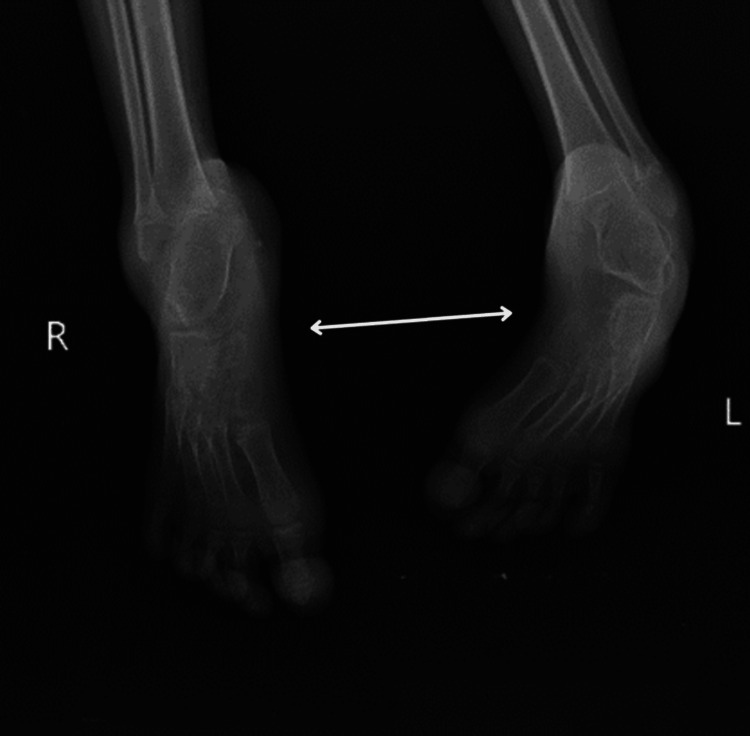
X-ray showing visible deformity at the ankle joint where the foot is in fixed plantar flexion, the longitudinal arch of the foot is exaggerated, and the foot is inverted and adducted at the midtarsal joint
